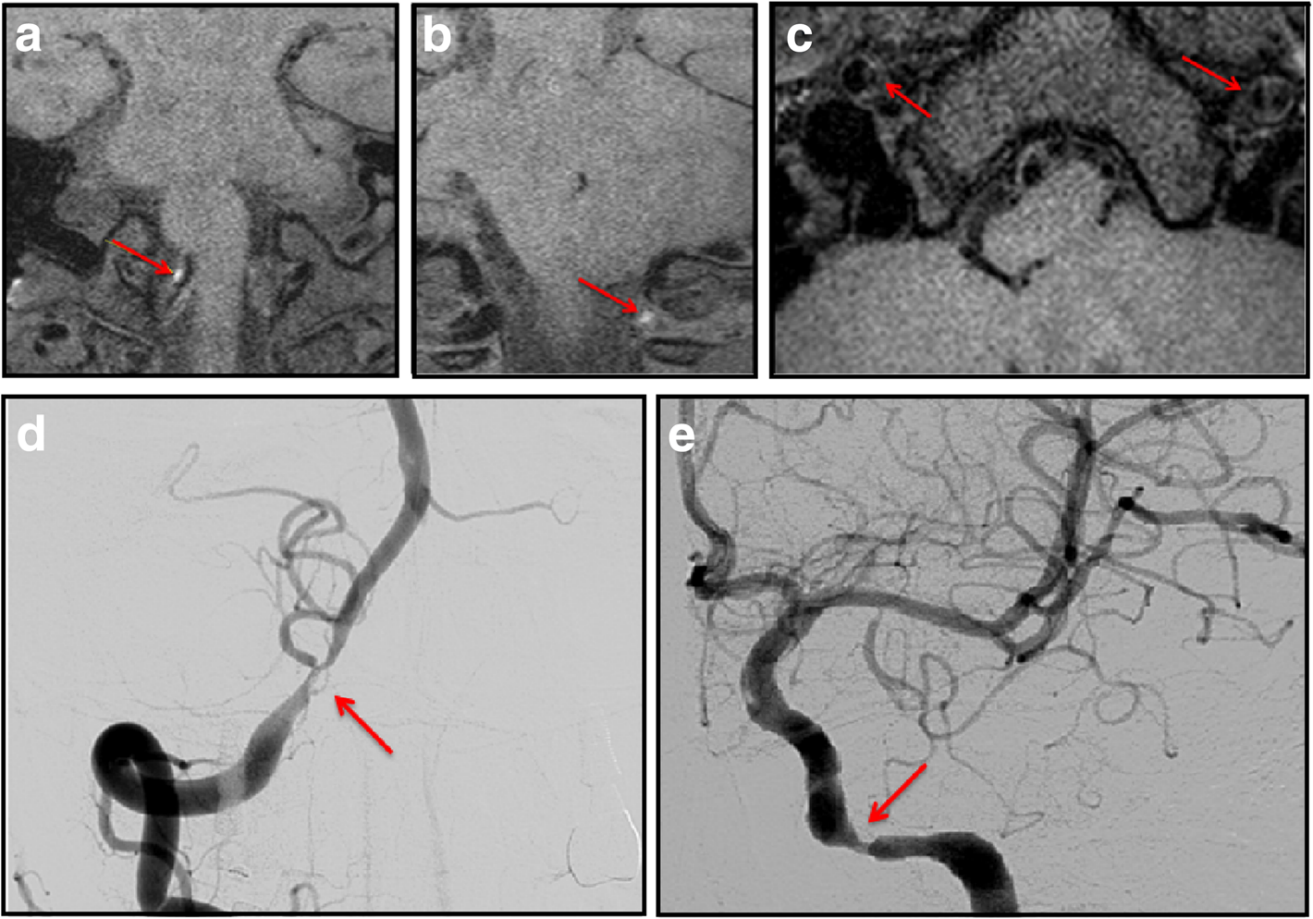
High-resolution MRI showing intramural hematoma at right (a; coronal image) and left vertebral arteries (b; coronal image) and dissecting flap in bilateral distal internal carotid arteries (c; axial image) Digital subtraction angiography shows severe stenosis at right vertebral artery (d) and left distal internal carotid artery (e)
